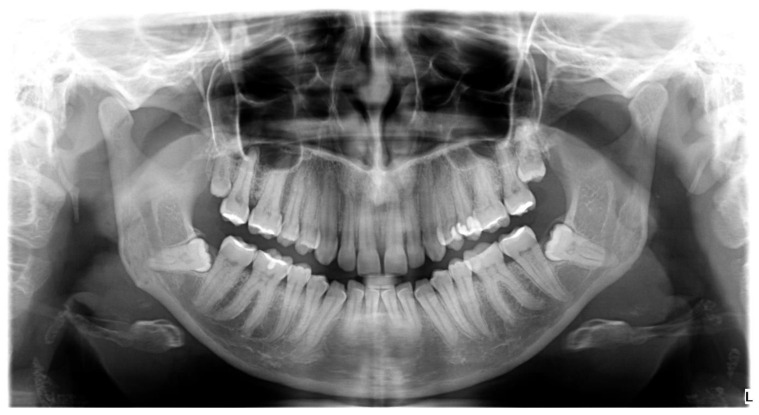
Impaction of four third molars on orthopantomogram (OPG).
